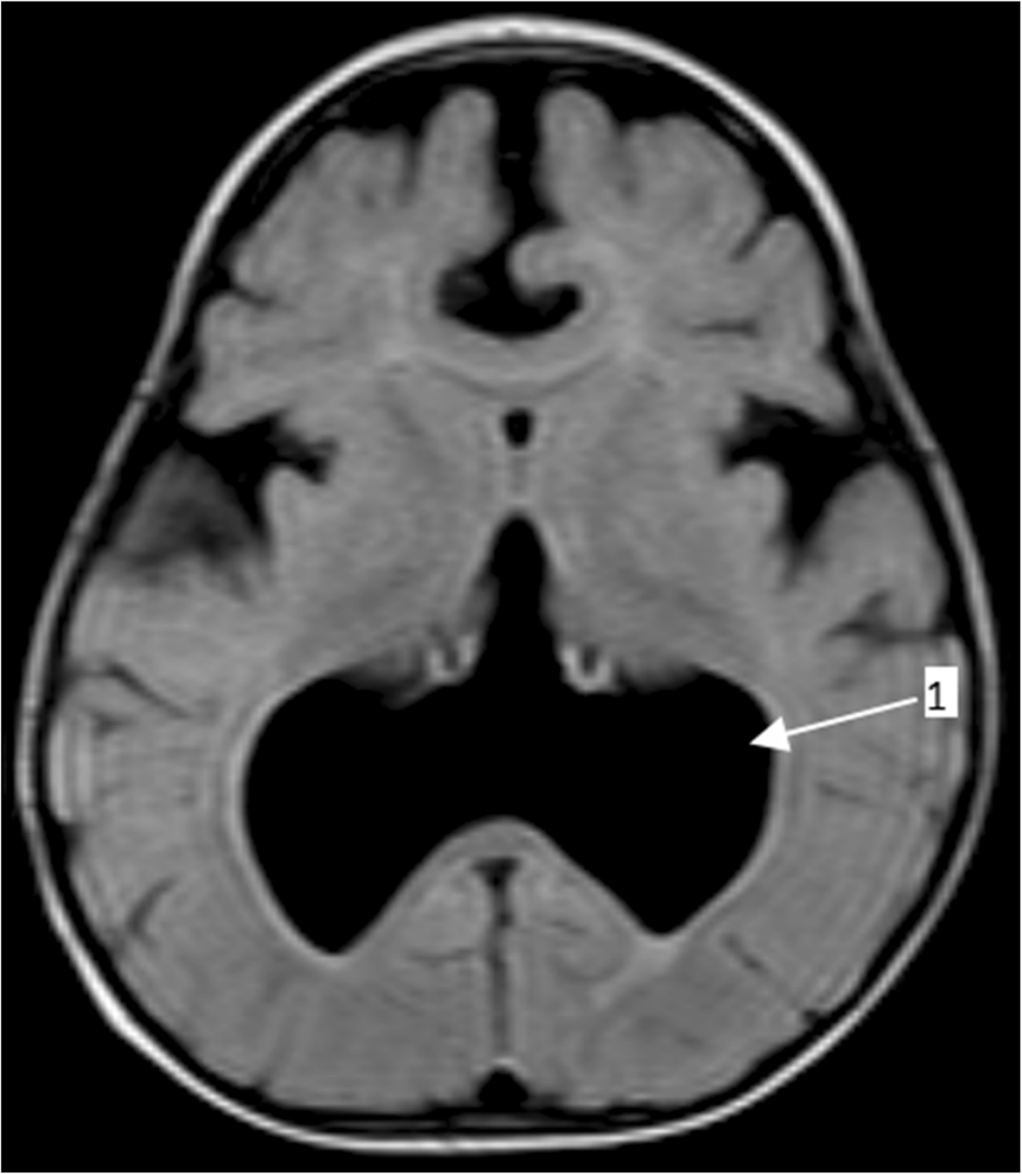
Brain magnetic resonance imaging (fluid-attenuated inversion recovery) displaying a mono ventricle with partially developed temporal and occipital horns (*arrow 1*)
